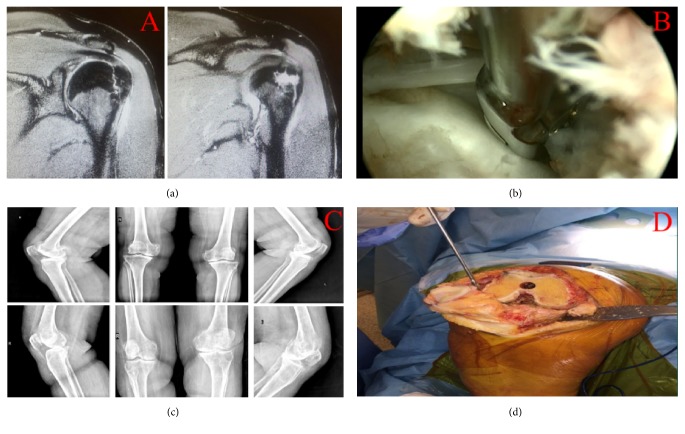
(a) Coronal MRI view of shoulder. (b) Arthroscopic view of shoulder. (c) X-rays of patients that have grade-IV gonarthrosis. (d) A view of knee arthroplasty surgery.
